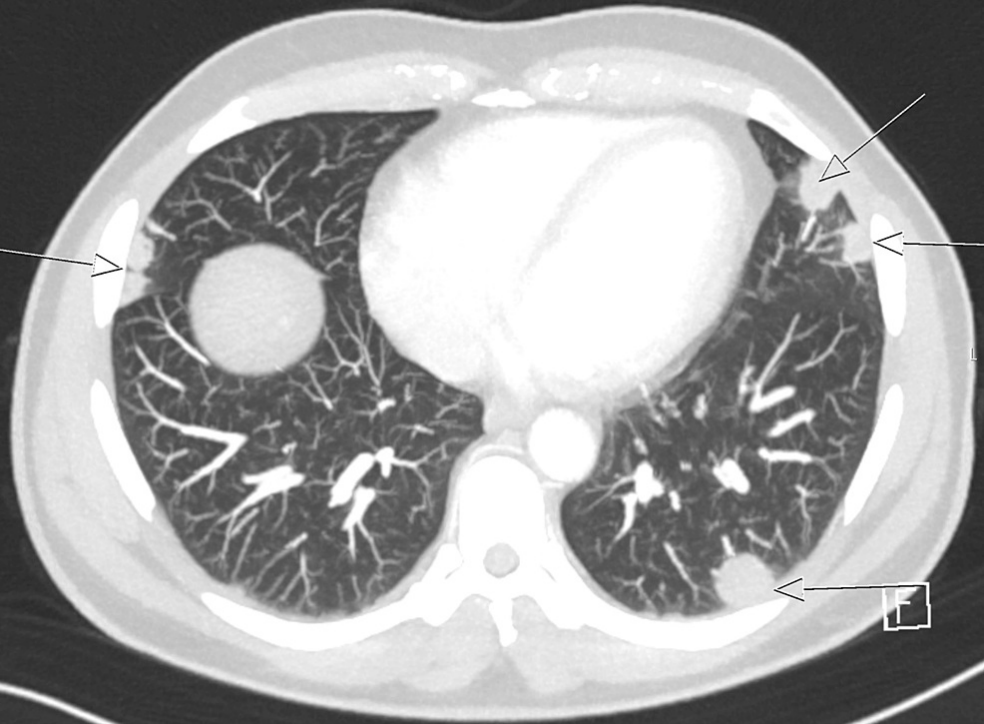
CT scan of the chest at hospitalization Transversal image showing labeled bipulmonal pleural-based opacities with the largest lesion up to 21 mm in diameter
